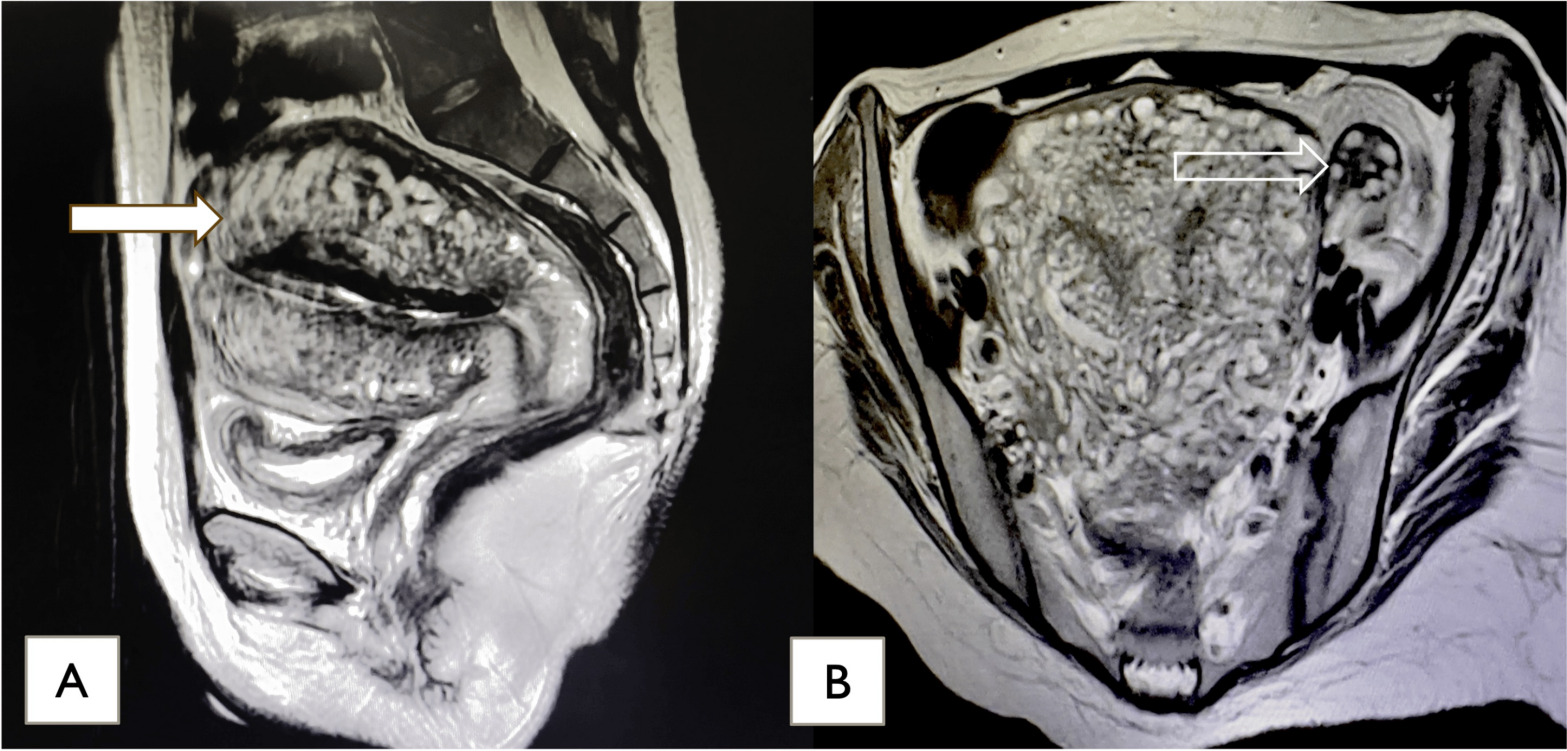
Pelvic MRI: (A) Sagittal view showing large hypervascularized uterus (post partum) marked by the arrow; (B) Axial view showing left ovary measuring 1.8x1.1x2.3 cm with multiple peripheral follicles (hollow arrow) and right ovary measuring 1.4x2.6x1.6 cm normal signal intensity and multiple peripheral relocated follicles. No adnexal mass could be seen.
